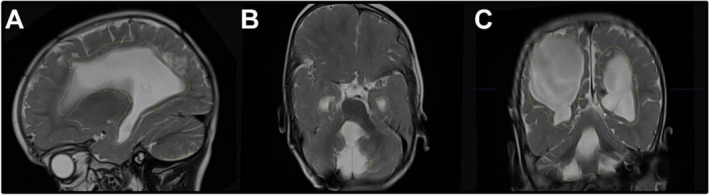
Screenshots of an iplan cranial 3.0 segmentation of the preoperative ventricle volume in comparison to an early control MRI objectifying the reduction of the ventricle size.
